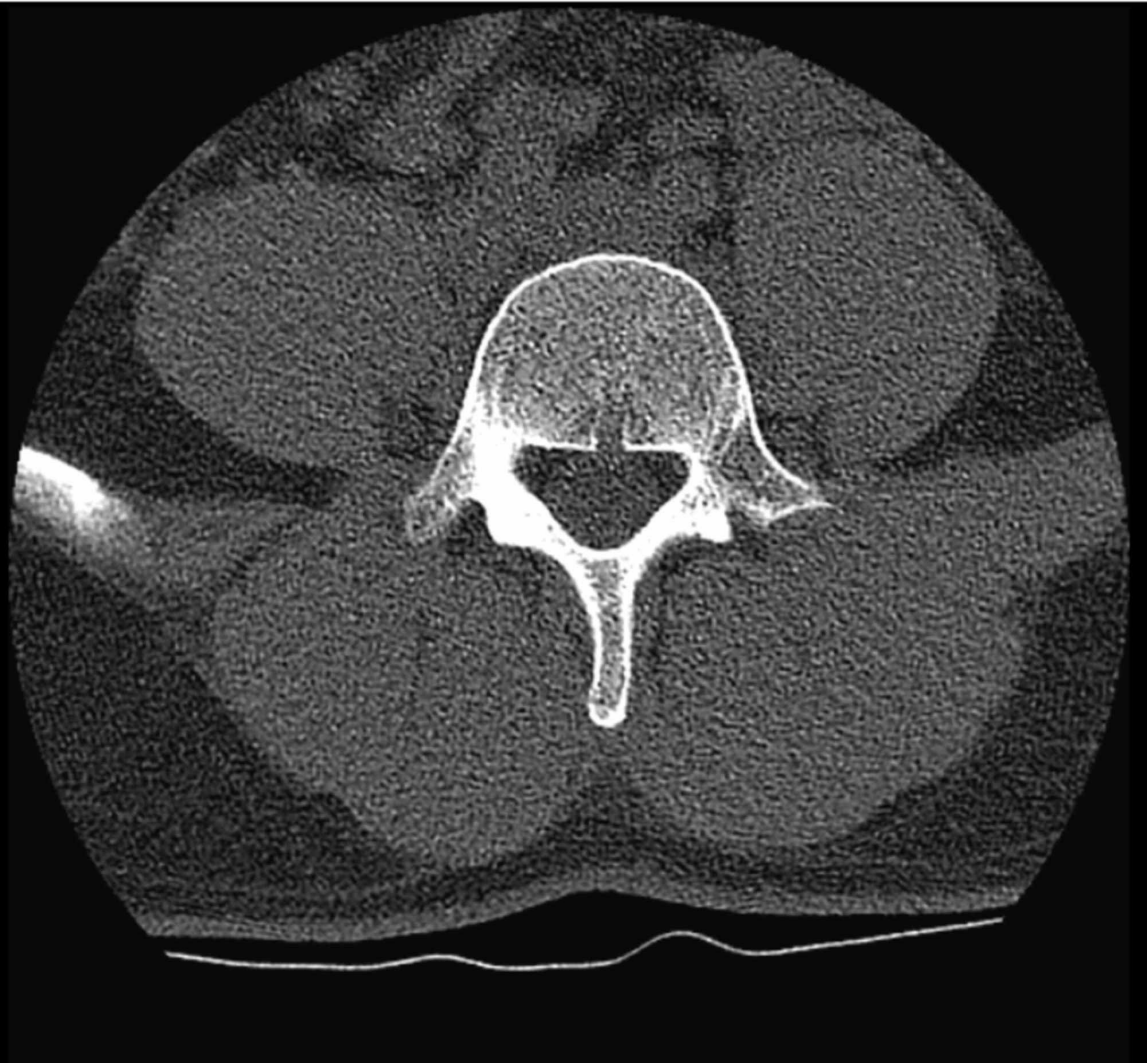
Computed tomography of the lumbar spine
